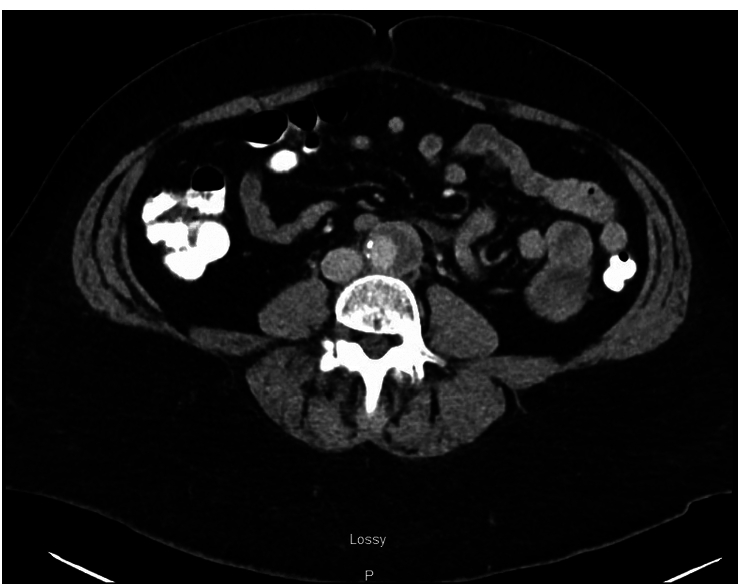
Computed tomography angiogram of the abdomen and pelvis showing circumferential soft tissue thickening in the distal aorta, suggestive of aortitis.
